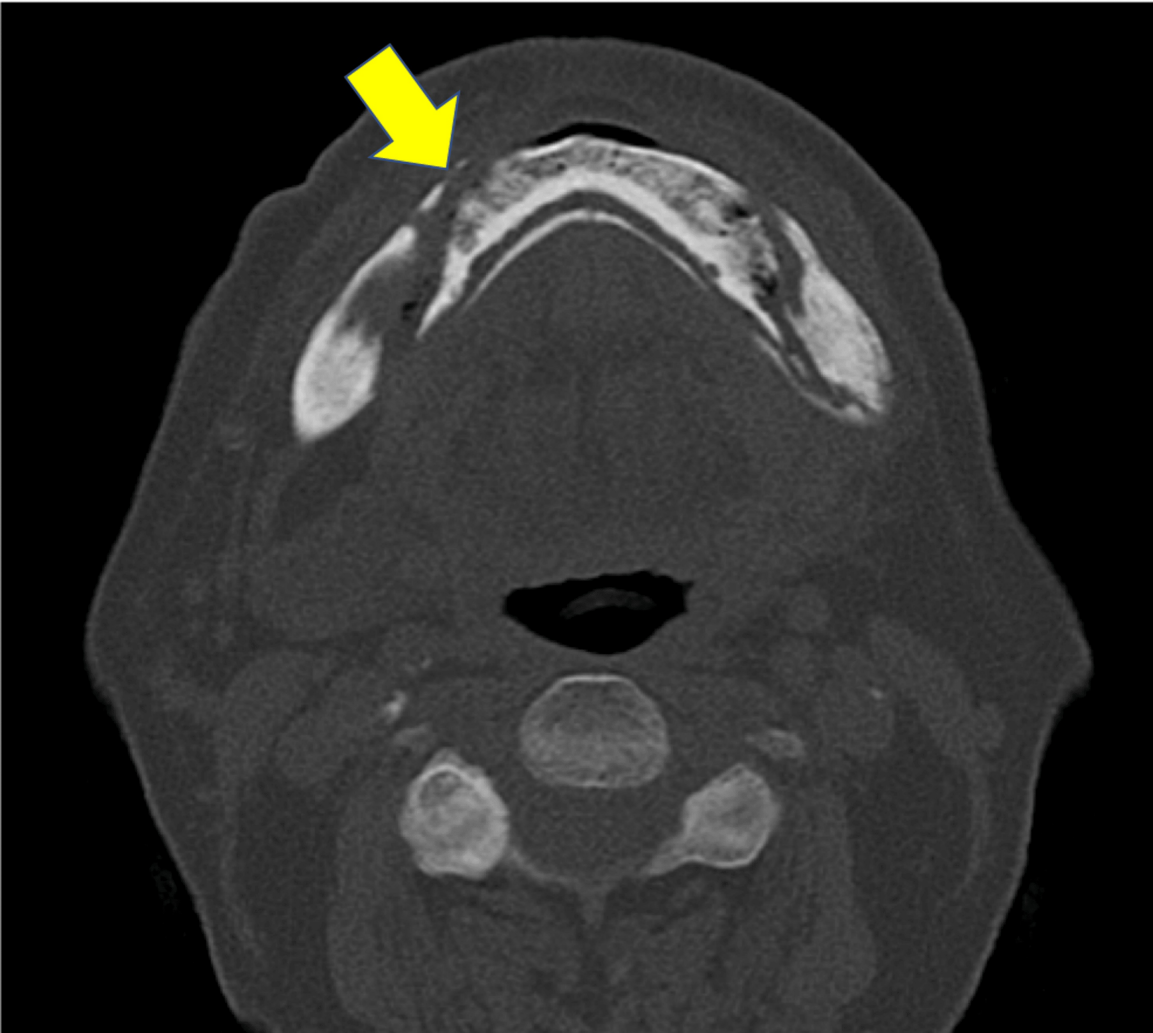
Radiographic finding after HBO treatment. The axial MDCT image showed increased separation of the sequestrum compared to the initial one (the yellow arrow indicates signs of sequestrum separation). MDCT, multidetector computed tomography; HBO, hyperbaric oxygen
